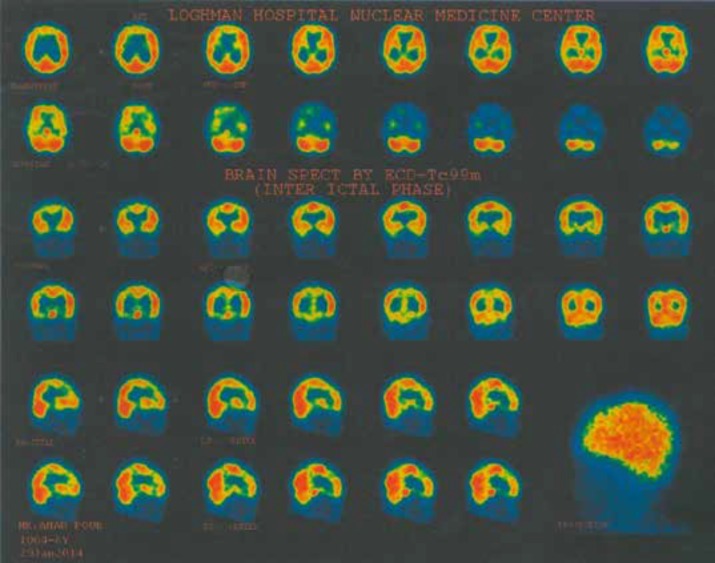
Brain perfusion SPECT with decreased perfusion in left centrotemporal lobe
